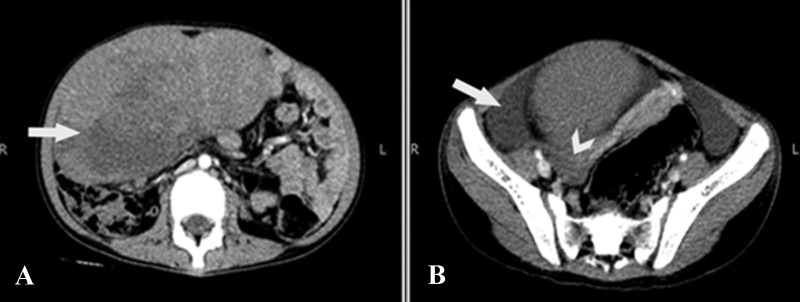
Computed tomography of the abdomen and pelvis axial sections A) Large right-sided lobulated pelvic mass with central areas of low attenuation suggestive of necrosis (arrow). B) There was free fluid adjacent to the lesion (arrow). The right ovary was separately visualised and appears normal (arrowhead).
